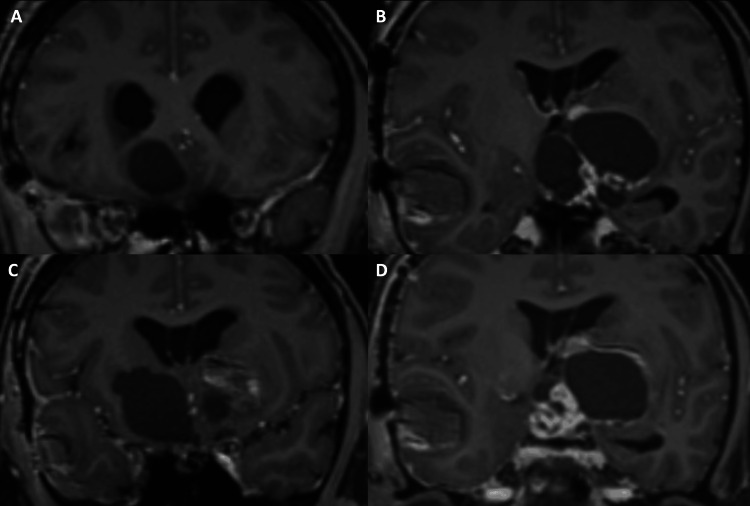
Multiple coronal sections of the T1-weighted images obtained post-gadolinium administration demonstrate the sellar-suprasellar solid-cystic lesion exhibiting mass effect on the right inferior frontal lobe (A), right basal ganglia (B, C), left basal ganglia (B, D) and left medial temporal lobe (B, D).
